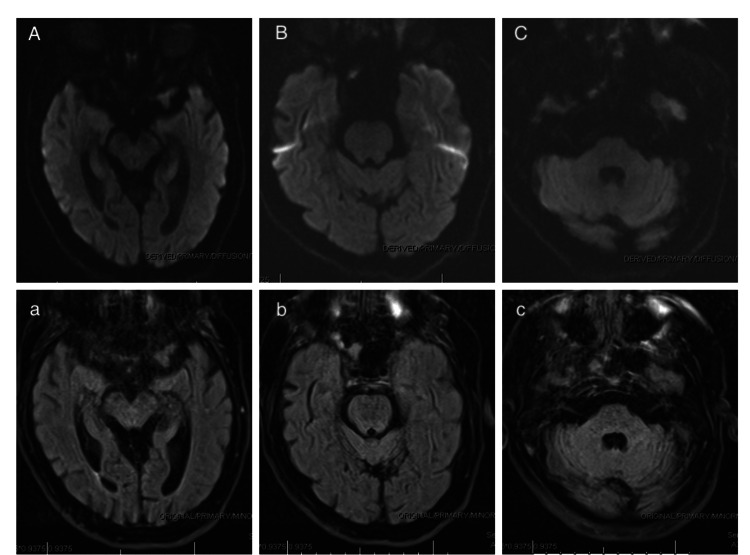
MRI DWI Upper A, B, C: DWI, lower a. b. c: FLAIR
